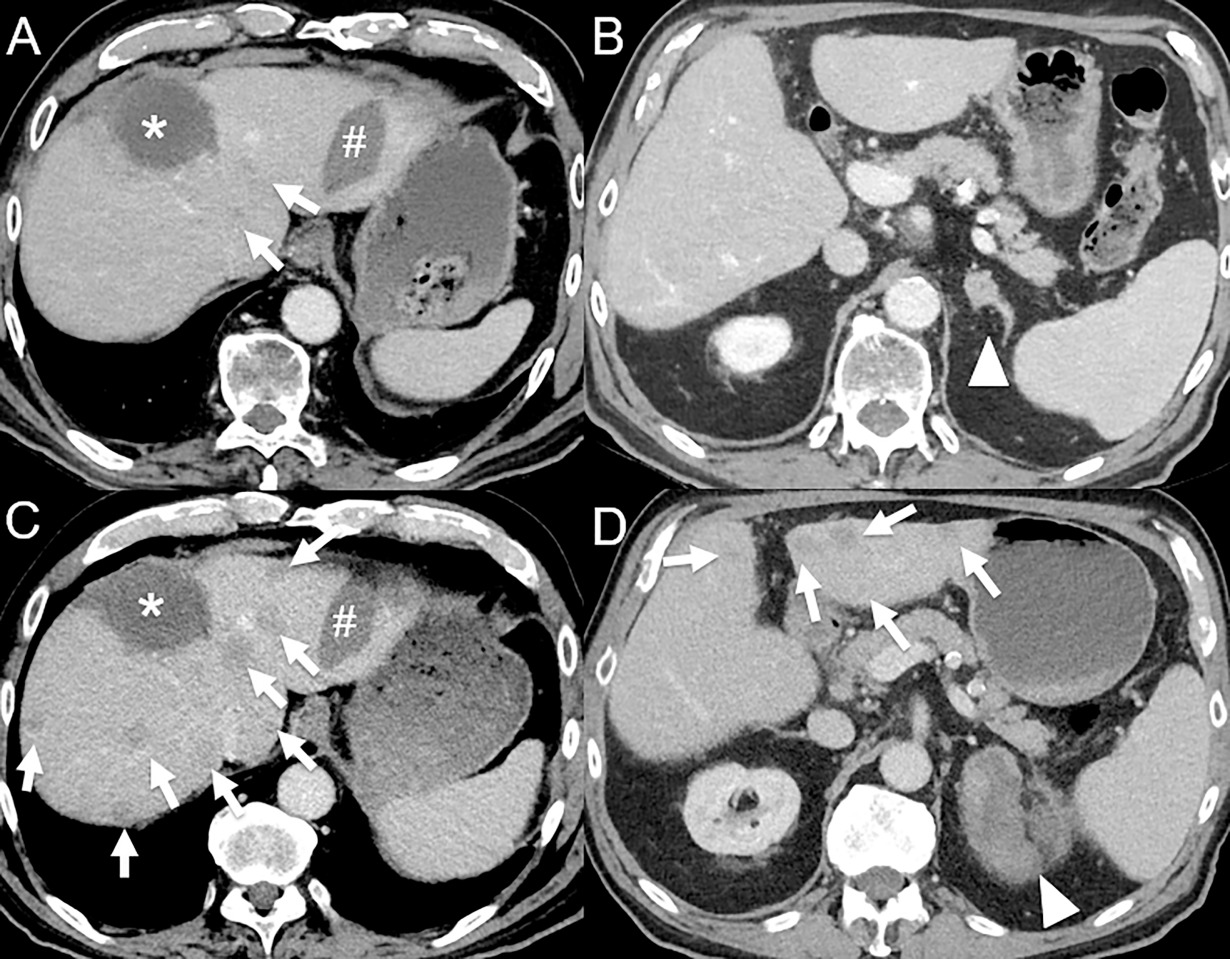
Hyperprogression in an 82-year-old male with hepatocellular carcinoma initially treated by selective internal radiation therapy and radiofrequency ablation who develop metastatic disease 11 months post-therapy (bone, left adrenal gland and lung). (A) Baseline contrast-enhanced CT at the portal venous phase shows necrotic lesion treated by selective internal radiation therapy (*) and a radiofrequency ablation scar (#), with 2 small hypodense lesions (arrows). (B) A left adrenal gland metastasis is also seen (arrowhead). The patient was given atezolizumab (anti-PD-L1) and bevacizumab (anti-VEGF). 1-month follow-up contrast-enhanced CT at the portal venous phase shows dramatic disease progression with the appearance of multiple cancer lesions [(C, D), arrows] and marked increase in the left adrenal gland metastasis [(D), arrowhead].
